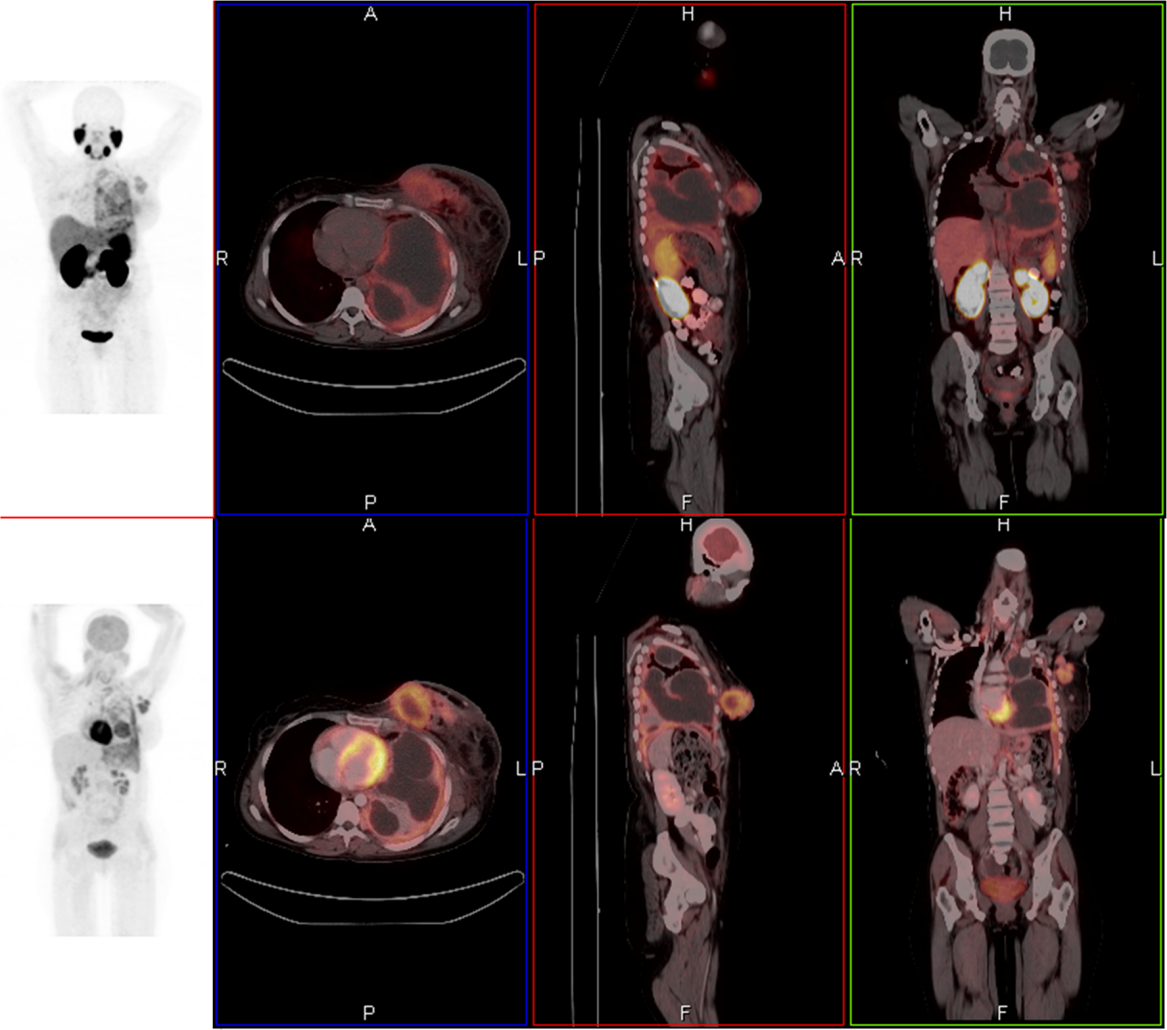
A 42-year-old female with metastatic breast carcinoma who underwent ^68^Ga-PSMA and ^18^F-FDG PET/CT. Axial, coronal, and sagittal fused ^68^Ga-PSMA PET/CT images demonstrated primary left breast cancer, axillary nodal and left pleural metastases (a). Avidity is slightly intense on ^18^F-FDG PET/CT images (b). Maximum-intensity-projection PET gives overview of all lesions (c, d)
